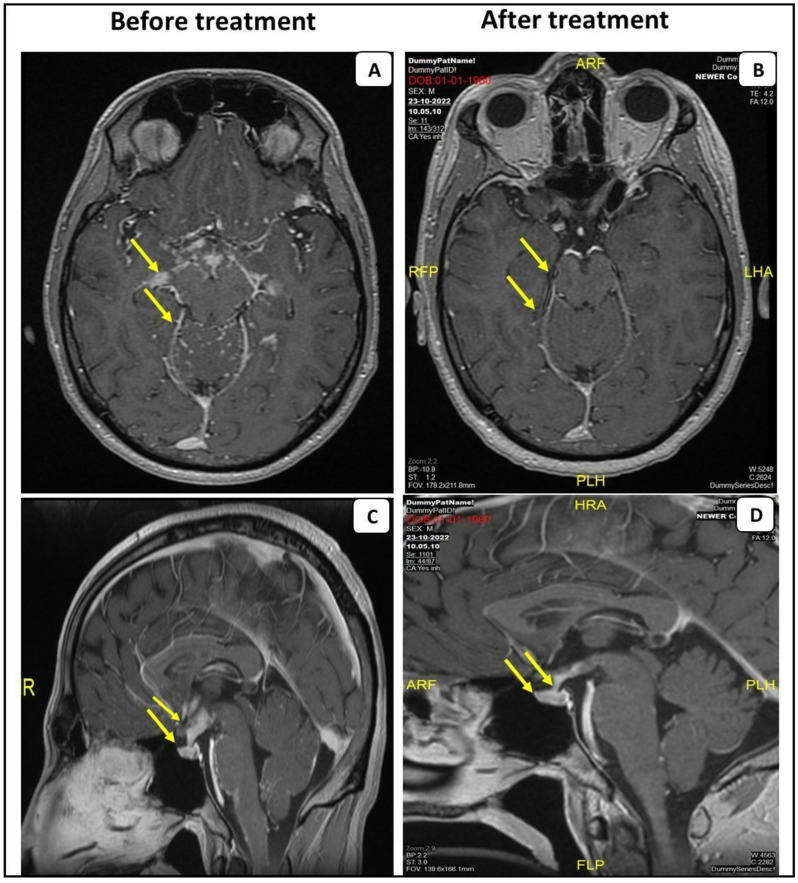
MRI scans with contrast of the first patient before and after the anti-TNF-α treatment. Post-gadolinium T1 MRI from the patient with probable NS, demonstrating leptomeningeal enhancement predominantly affecting the basal cistern of the brain and leptomeningeal spread within the folia of the cerebellum (A). Sagittal post-gadolinium T1 MRI demonstrates abnormal contrast enhancement and swelling of the pituitary gland (arrowhead) (C). Anti-TNF-α treatment resolved these findings (B,D).
